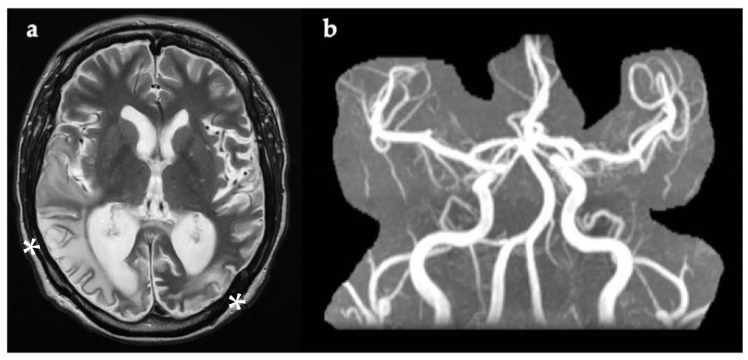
(a) Axial brain MRI image taken four days after symptom onset, showing multiple areas of white matter and cortical increased T2w signal within the posterior cerebral hemispheres (white asterix). DWI is not available. (b) Normal MRA with no evidence of narrowing of blood vessels, as usually present in CNS vasculitis. DWI, diffusion-weighted imaging; MRA, magnetic resonance angiography; T2w, T2-weighted imaging.
